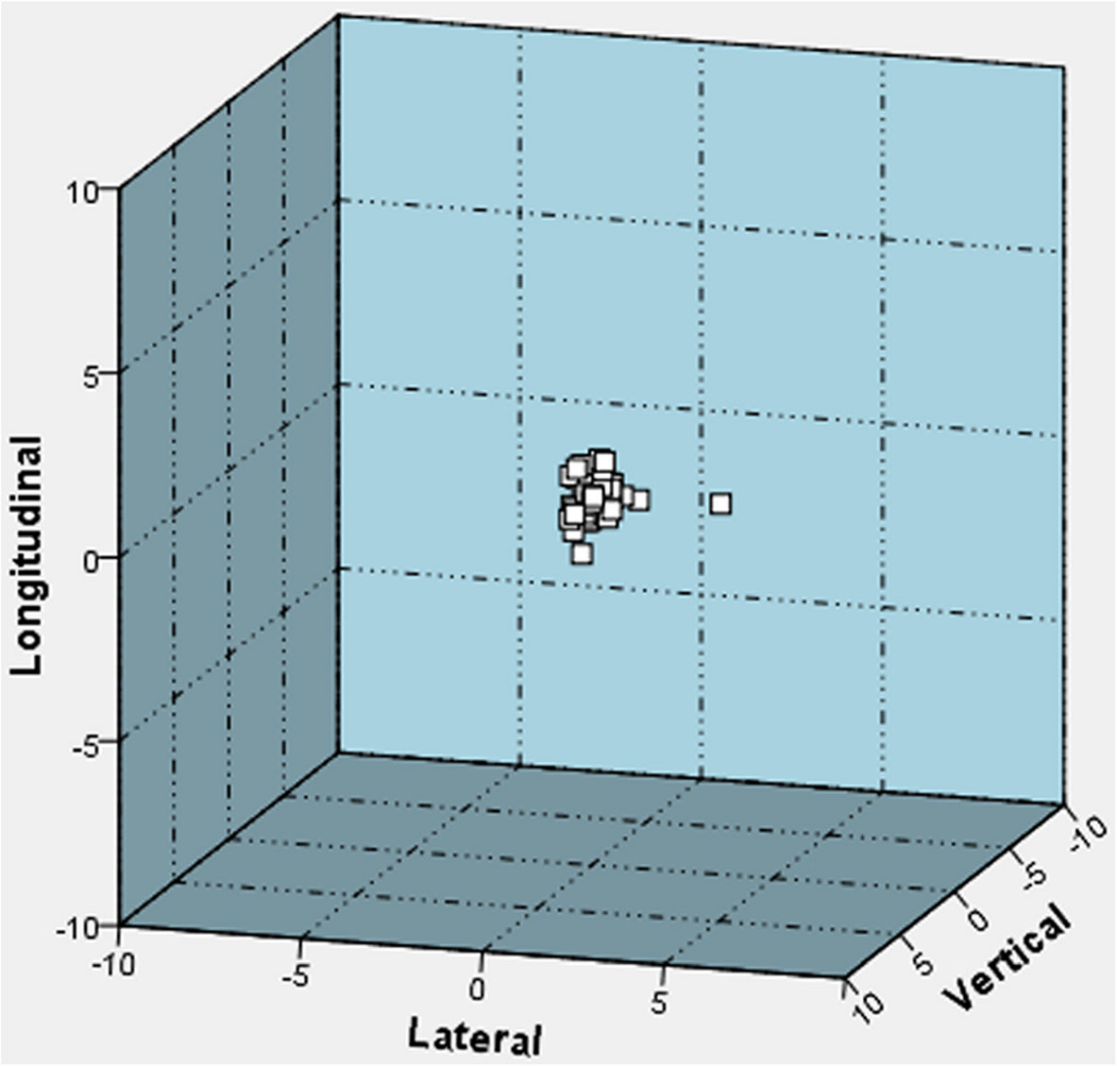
Online adjustments after cone beam computed tomography scans with abdominal compression [cm]
